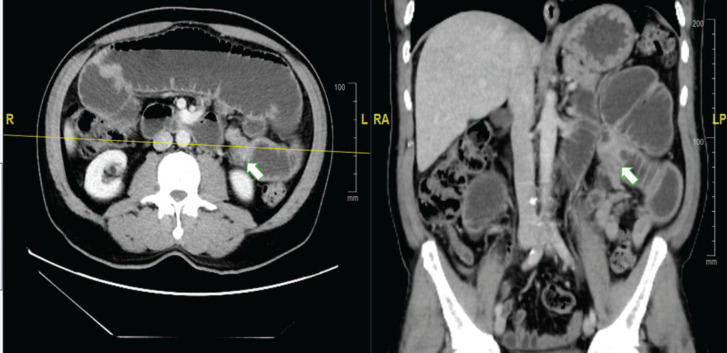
Abdominal CT scan with contrast. (a): Axial section. (b): Coronal section. The arrow indicates the point of tumorous intestinal stenosis, causing retrograde dilation of the proximal small intestinal loops.
